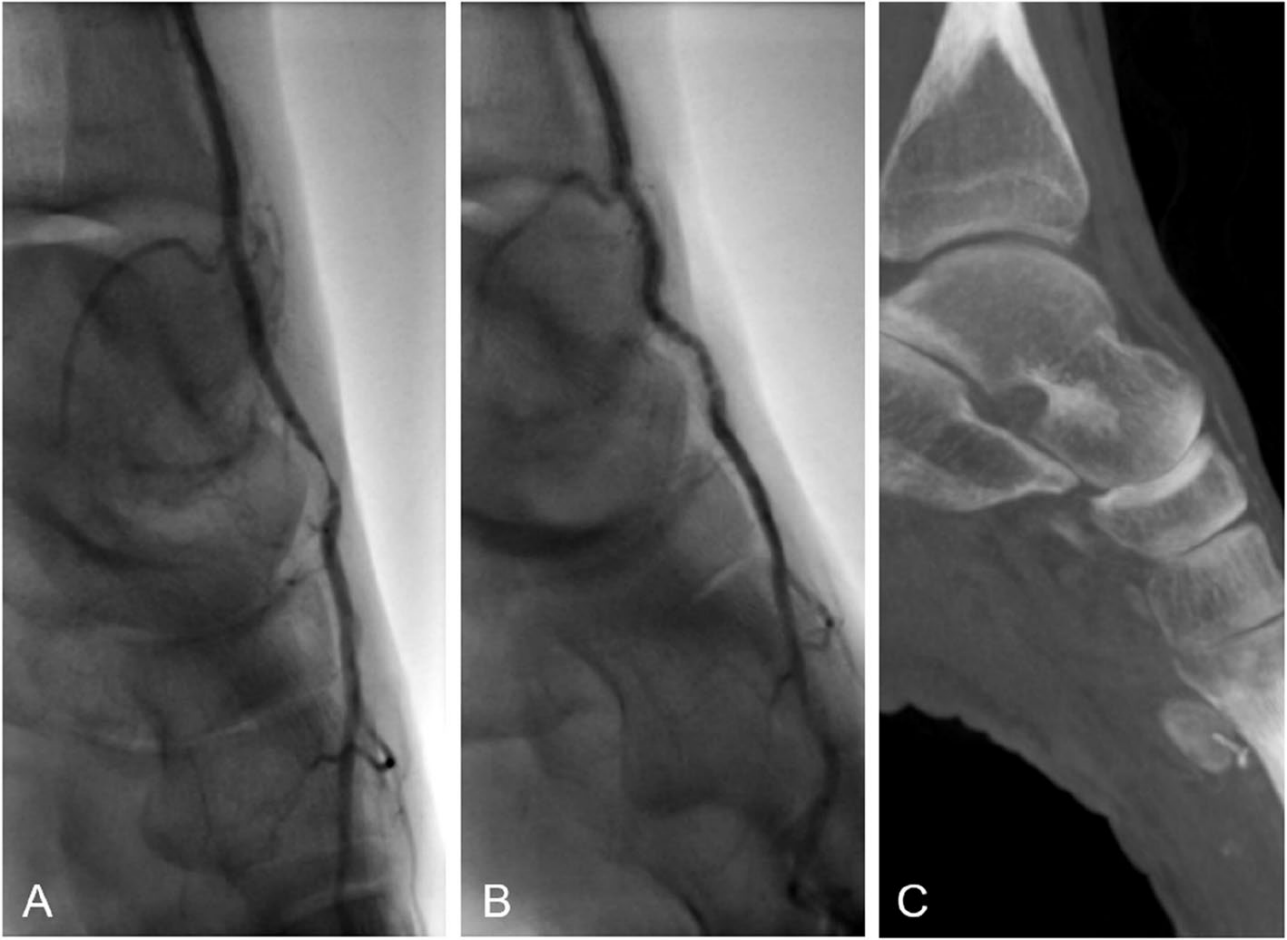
Dynamic fluoroscopic angiography imaging depicting DPA compression. In image A, an angiogram in spontaneous normal position of the left foot is seen with filiform stenosis of the DPA at the anterior cranial part of the talus. In image B, dynamic angiography in dorsal extension in the ankle joint shows complete disappearance of the stenosis, confirming functional vascular compression. Image C shows cone-beam CT imaging of the left foot depicting the talonavicular subluxation leading to functional intermittent bony compression of the DPA
